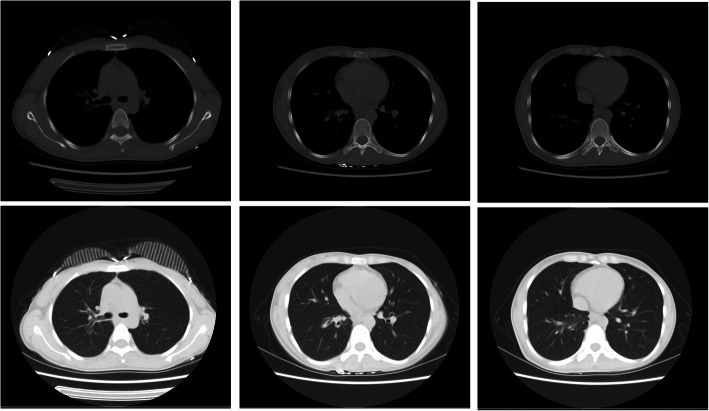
Chest CT of the patient. The right upper lobe had minute nodules; the right lower lobe had proximal segmental lung insufficiency; the right paraspinal pleura was slightly thickened; the mediastinal lymph nodes were increased; and the wall of the esophagus was limited and slightly thickened. No swollen lymph nodes on cervical region and bilateral supraclavicular fossa is evident
